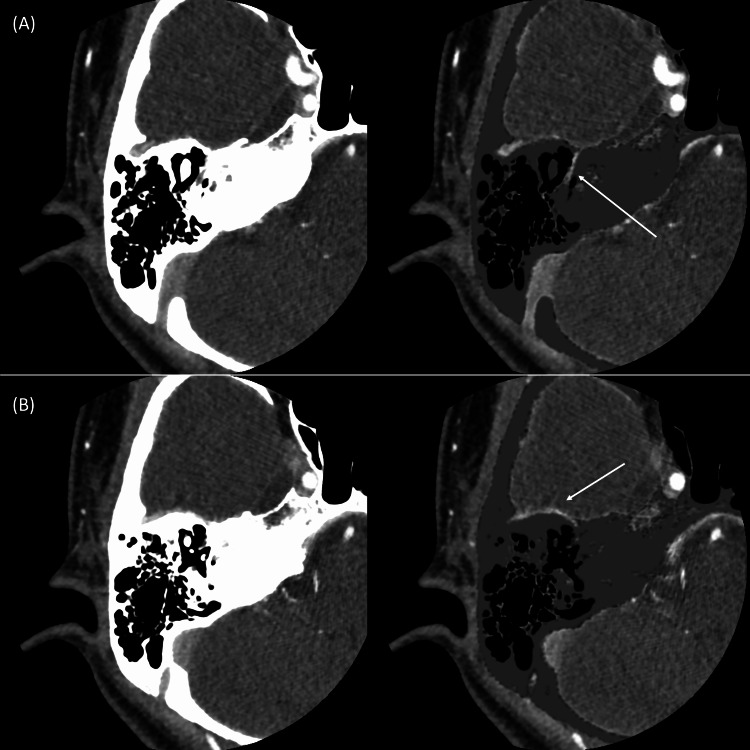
Subtraction images of right temporal bone CT. The right side is a subtraction image and the left side is an image of the soft tissue window. The slices are the same on both sides. A vascular structure runs around the stapes (A: white arrow) and is connected to the middle meningeal artery (B: white arrow).
